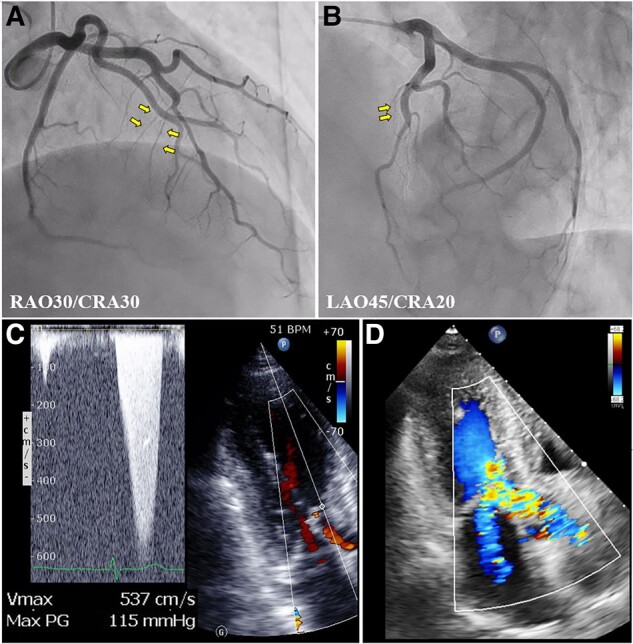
Pre-operative evaluation of the left ventricular outflow tract obstruction and systolic anterior motion–related mitral regurgitation. (*A* and *B*) Initial coronary angiogram shows target septal branches (arrows). (*C* and *D*) Transthoracic echocardiography showing a left ventricular outflow tract pressure gradient and mild systolic anterior motion–related mitral regurgitation before percutaneous alcohol septal ablation. CRA, cranial; LAO, left anterior oblique; RAO, right anterior oblique.
